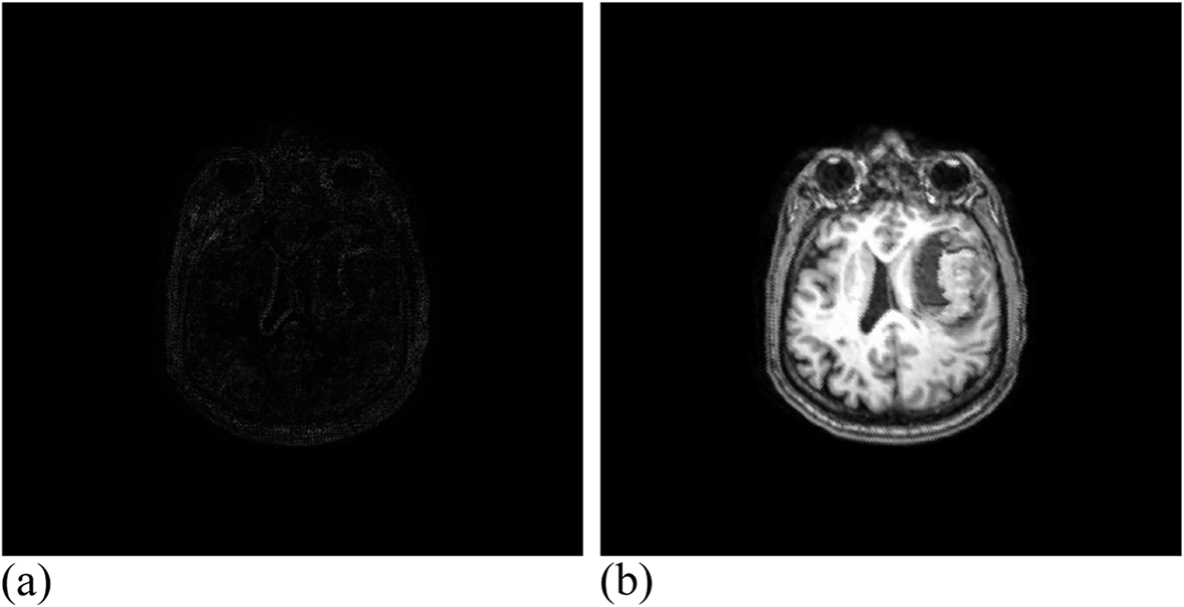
MRI data after registration. (a): Difference between MRI reference image and registration image; (b): MRI registration image
